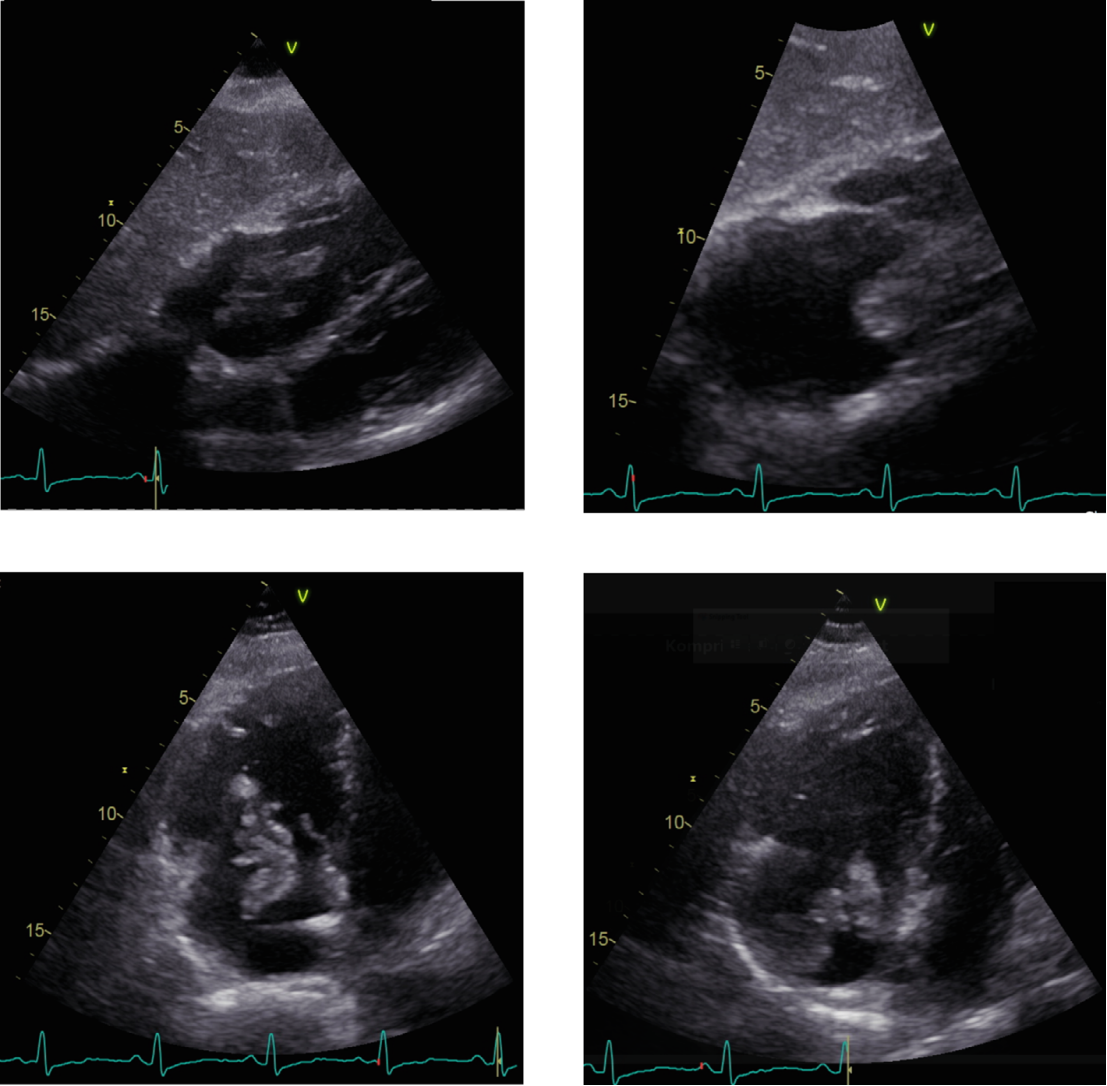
Subcostal and apical four‐chamber view of a mobile thrombus detected in the right heart cavities, originating most likely on the right atrial roof.
